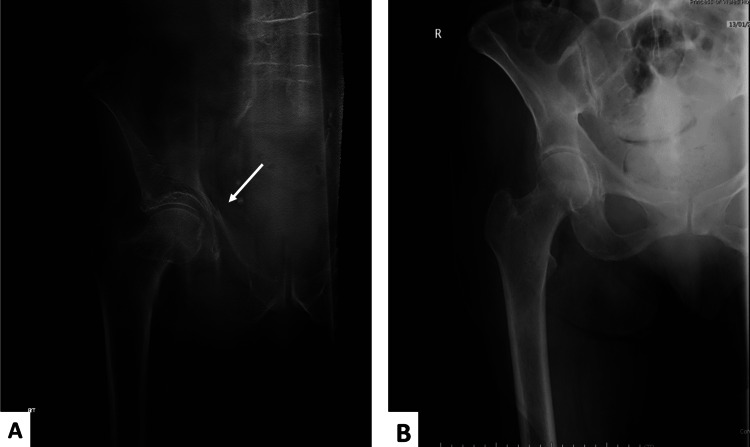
(A) A 97-year-old male patient tripped and presented with pain and a non-weight-bearing right hip. The patient had plain radiographs, which did not show an obvious fracture. The tomogram showed an acetabular fracture (arrow). (B) An 80-year-old female patient tripped and presented with left hip pain and non-weight-bearing. Due to patient claustrophobia, MRI imaging was abandoned, and instead, tomogram imaging was used, which did not show any fracture.
